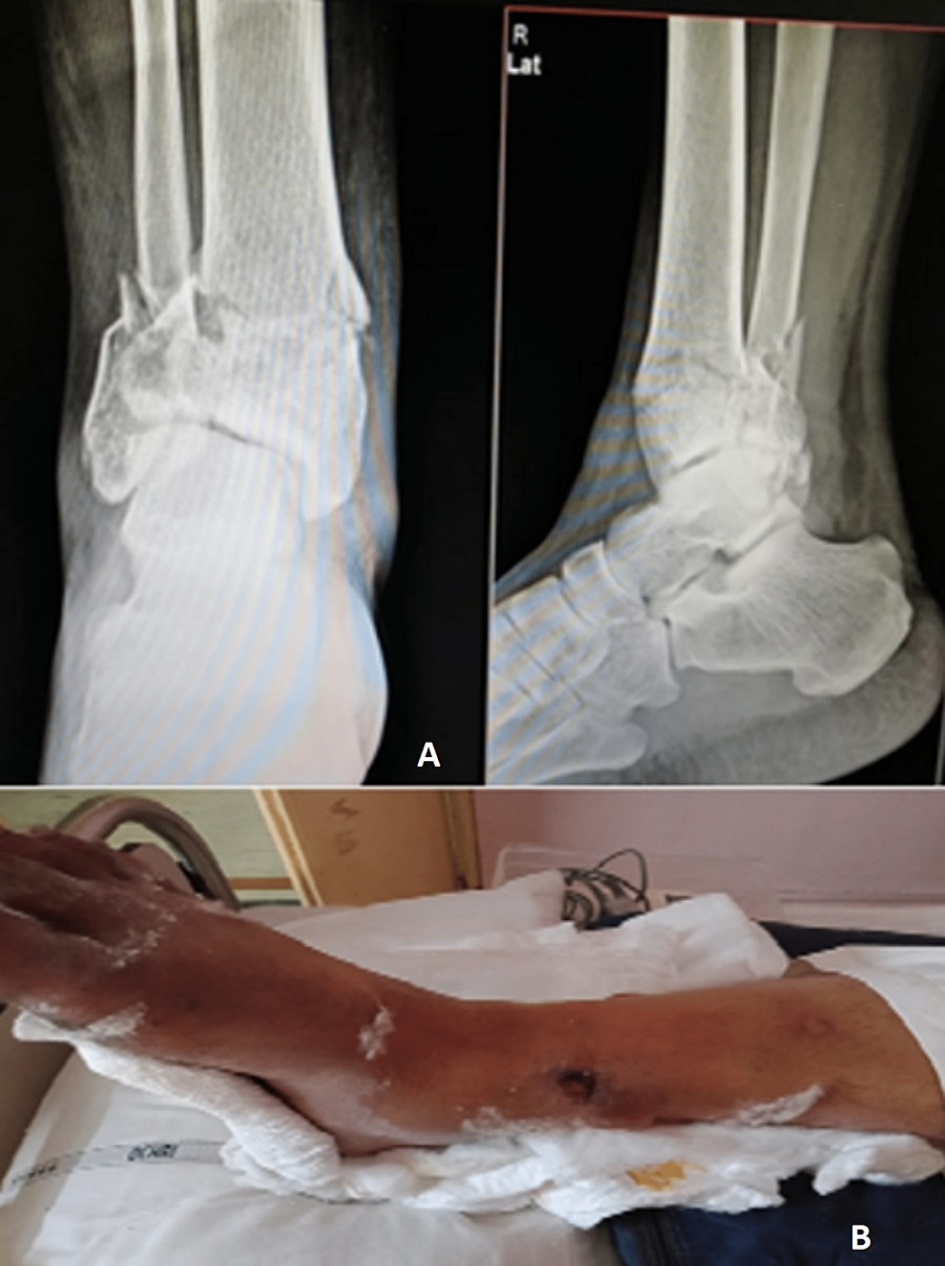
Pre-operative X-rays and clinical image A: Pre-operative X-rays in anteroposterior and lateral views B: Pre-operative clinical image
